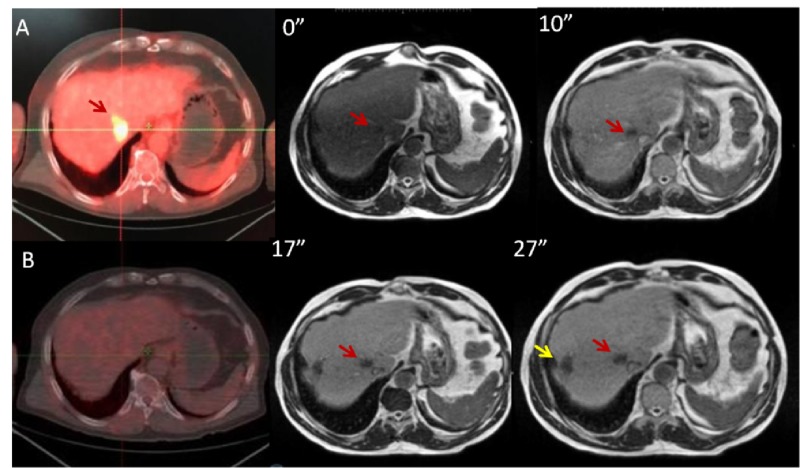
PET-CT and MRI scans with gadoxetate (A) PET-CT scan before treatment, (B) 3rd month follow-up PET-CT scan after radiosurgery, 0-10-17-27 minutes of gadoxetate injection: Red arrow denotes tumoral target, yellow arrow denotes previous treated area with interventional radiology.
